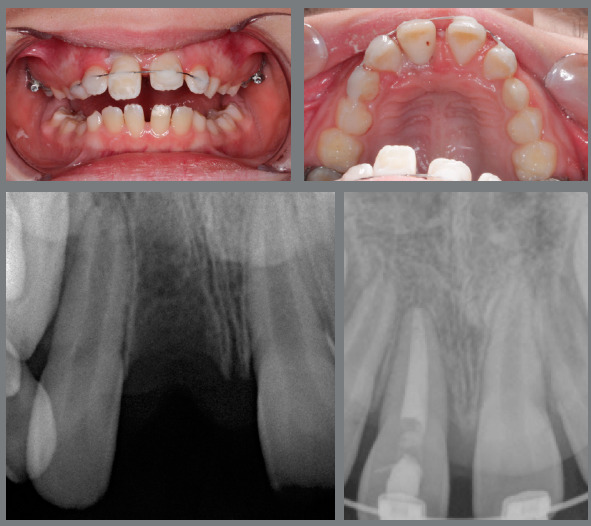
Clinical and radiographic aspects after reimplantation.
